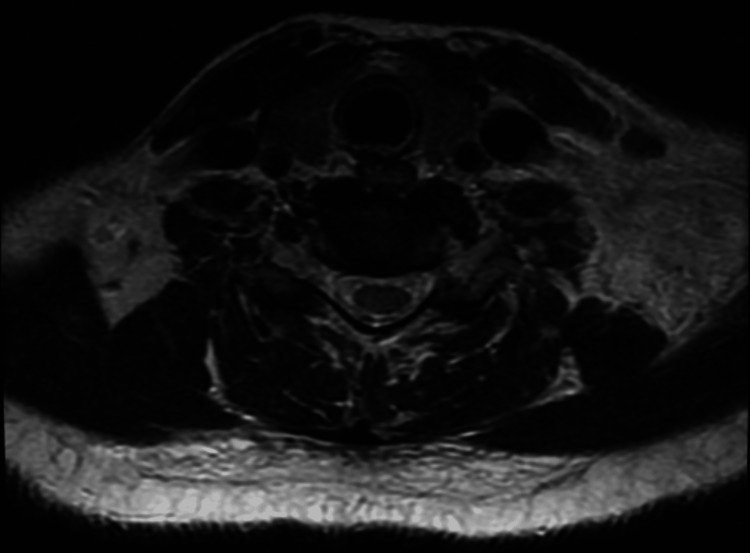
Transverse head and neck T2 magnetic resonance imaging Transverse head and neck T2 magnetic resonance imaging demonstrating left shoulder and supraclavicular edema extending into the left paravertebral soft tissues, left scalene, and left trapezius.
